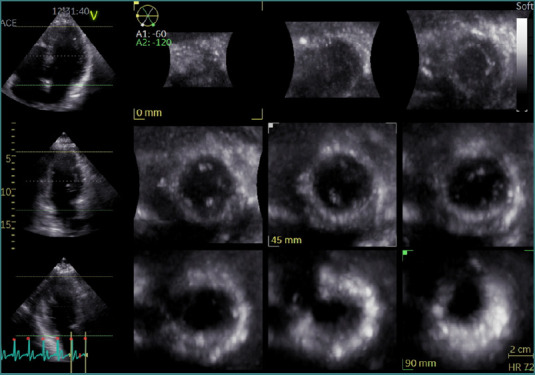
Acquiring a three-dimensional (3D) echocardiographic image of the left ventricle using a multi-beat full-volume technique
